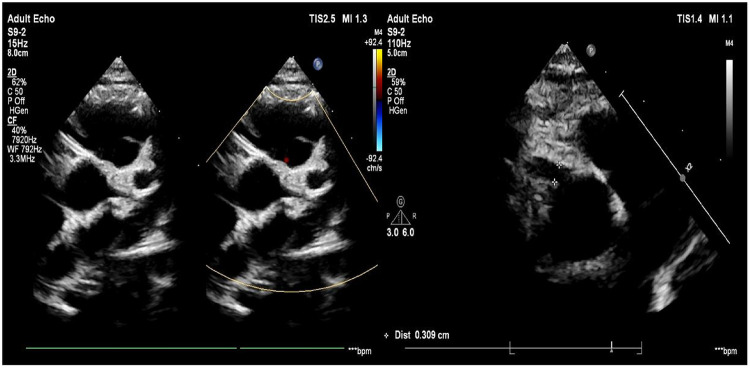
Echocardiography on the 24th day of hospitalization (left anterior descending coronary artery *Z*-score 10.51, right coronary artery *Z*-score 10.00). *Z*-scores calculated as per Figure 2.
